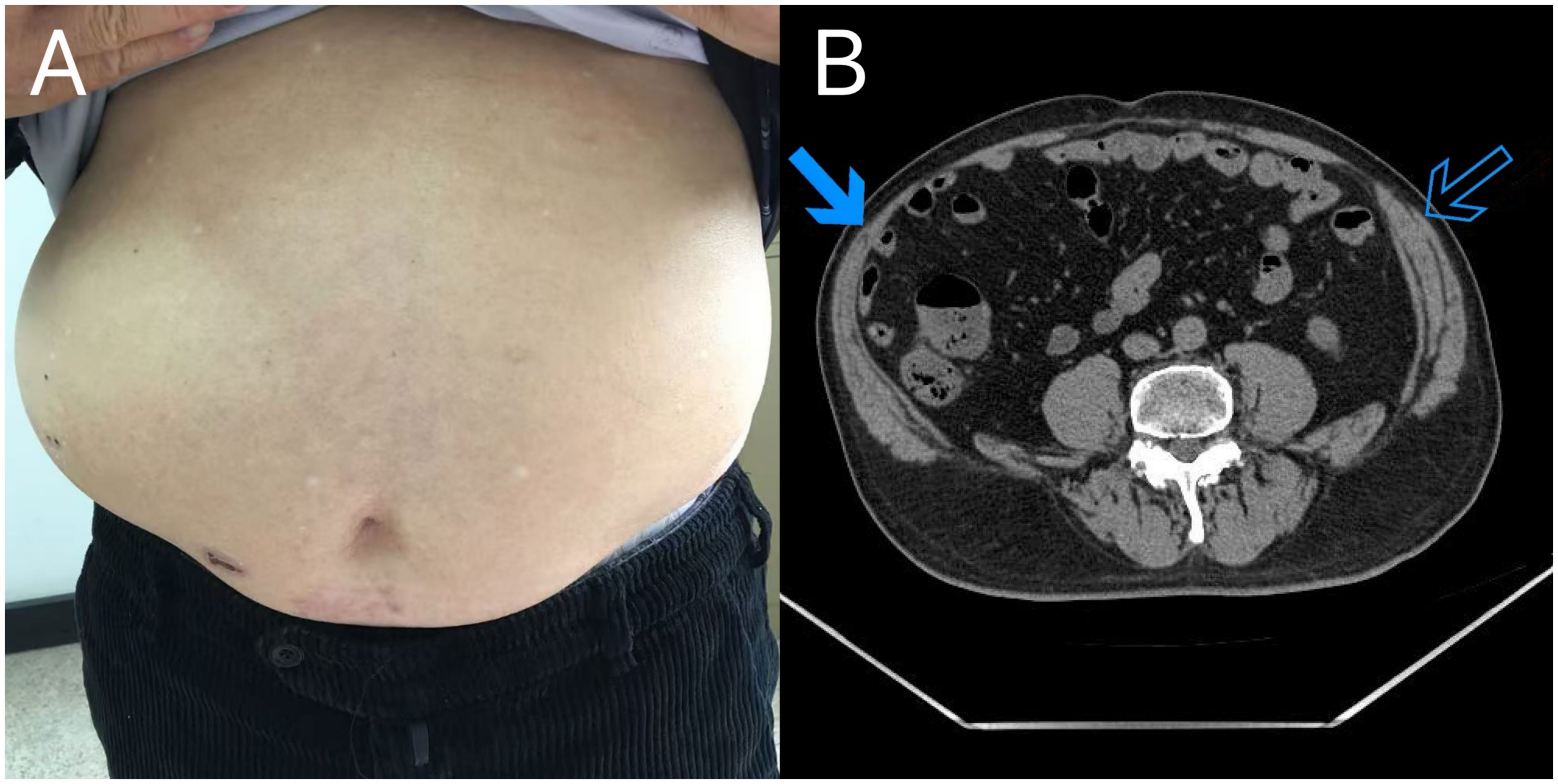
(A) Clinical photograph shows a right-sided abdominal wall bulge. The overlying crusts represent resolving herpes zoster vesicular lesions located in the same anatomical region. (B) Axial computed tomography image demonstrates ipsilateral thinning of the abdominal wall muscles on the affected side (solid arrow) compared with the contralateral side (open arrow), with no evidence of a fascial defect, true hernia, or other structural abdominal pathology.
